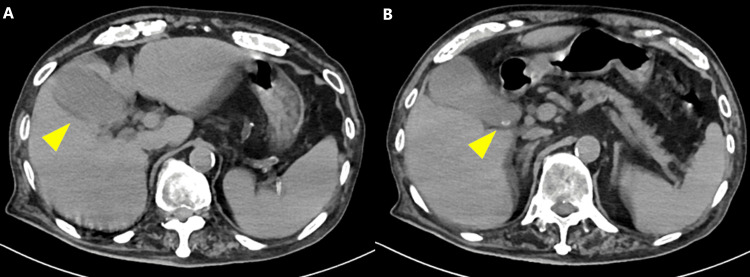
Abdominal non-contrast computed tomography on hospital day 15 (A) Persistent gallbladder distension with increased pericholecystic fat density, showing no significant change from the initial study. (B) A 6-mm gallstone at the gallbladder neck, unchanged from the initial study.
